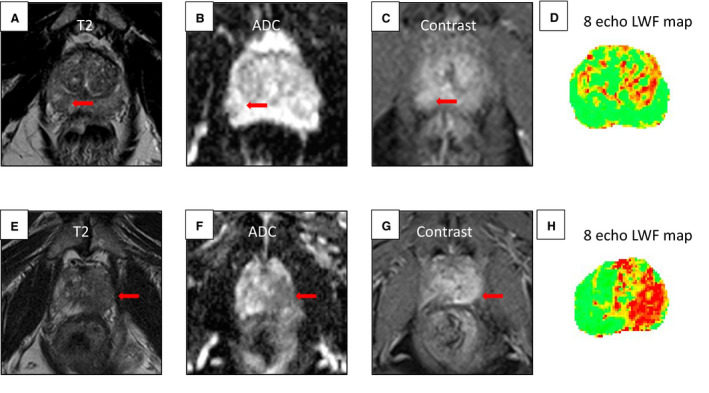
Comparison of conspicuous standard MRI lesions with luminal water fraction (LWF) imaging mapping and targeted biopsy. Lesion indicated by red arrow. Top row from left to right: 65‐year‐old man with PSA 9.9: (A) T2‐weighted MRI showing a region of lesion with reduced signal intensity right periphery. (B) ADC map showing reduced signal intensity in the lesion. (C) Dynamic contrast‐enhanced (DCE) image showing increased signal intensity at the lesion. A Likert score of 4 was given. (D) LWF imaging mapping, which does not indicate the presence of a lesion. Biopsy result: benign. Bottom row from left to right: 68‐year‐old man with PSA 14: (E) T2‐weighted MRI showing a region of reduced signal in the left peripheral zone extending to the transitional zone. (F) ADC mapping, showing reduced signal intensity at the peripheral lesion. (G) DCE imaging, showing lesion enhancement. A Likert score of 5 was given. (H) LWF mapping was positive at the lesion. Biopsy result: positive for cancer Gleason 4 + 3.
